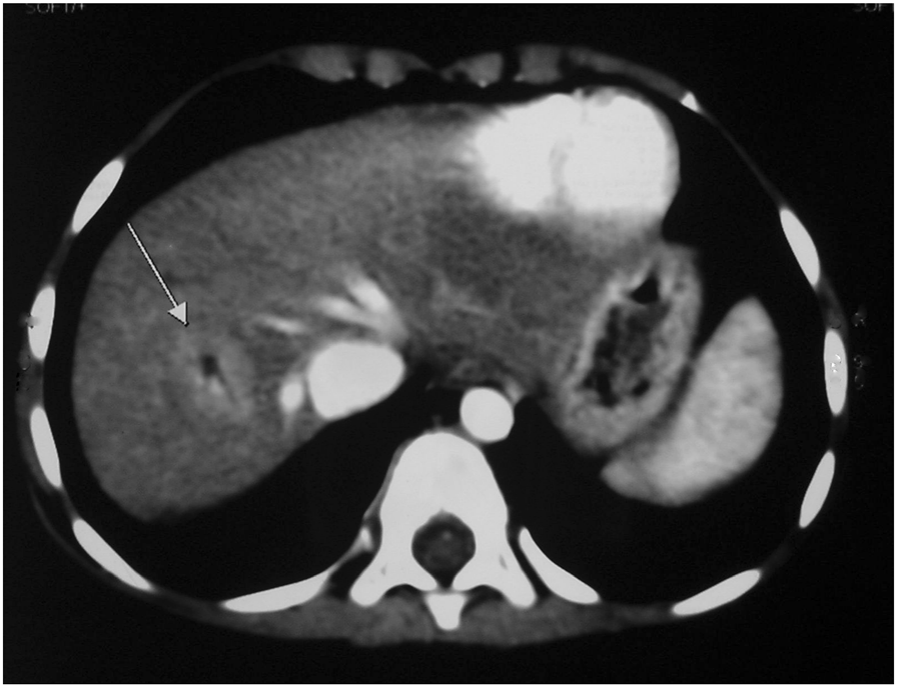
CT scan abdomen: Mass lesion in right lobe of liver (marked by white solid arrow).
